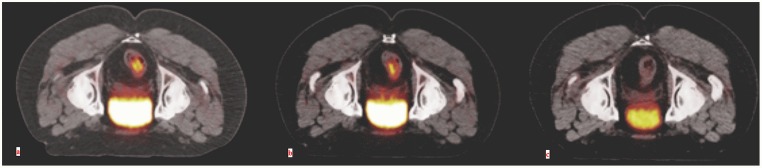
Sequential PET/CT studies in patient n. 2. Transaxial fused PET/CT images obtained in the prone position in the basal (a), early (b) and delayed (c) studies. A clear progressive reduction in FDG accumulation of the rectal lesion can be appreciated. This patient had a complete pathological response on pathological analysis (TRG1).
